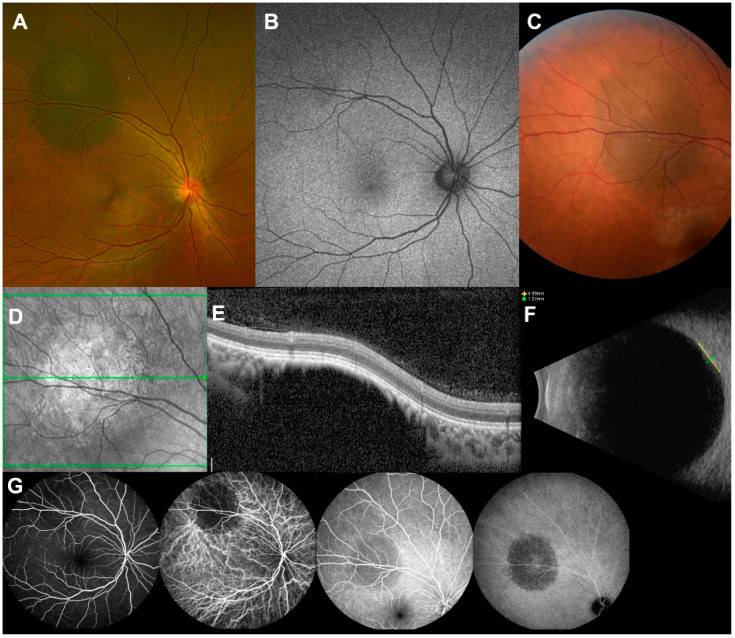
Multimodal imaging of a benign choroidal nevus of a Korean patient. A 21-year-old female patient was referred for an incidental finding of a choroidal tumor in the right eye. (A) Wide fundus photography shows a dark pigmented relatively flat 6.0 × 5.2 mm sized intraocular mass, 4.7 mm away from the optic disc and 3.4 mm away from the foveola. (B) Autofluorescence image shows a relatively isoautofluorescent mass at superior arcade. (C) Color fundus photography shows a greyish charcoal colored mass. (D) Near-infrared image shows a bright reflectance at the center of the mass. (E) Optical coherence tomography shows a hyporeflective intrachoroidal mass with 1105 μm thickness. Choriocapillaris over the mass seems compressed and thinned showing hyper-reflectance. (F) B-scan ultrasonography shows an echodense choroidal mass with 1.27 mm thickness. (G) Fluorescence angiography and indocyanine green angiography show a hypofluorescence at the early and late phase. With the diagnosis of a benign choroidal nevus with one risk factor (diameter over 5.0 mm), she was regularly followed-up under surveillance for malignant change.
